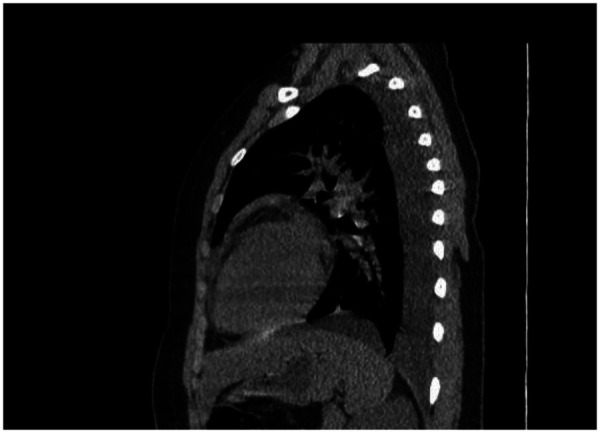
Non- contrast CT chest demonstrating bilateral pleural effusions and trace pericardial effusion at presentation.
